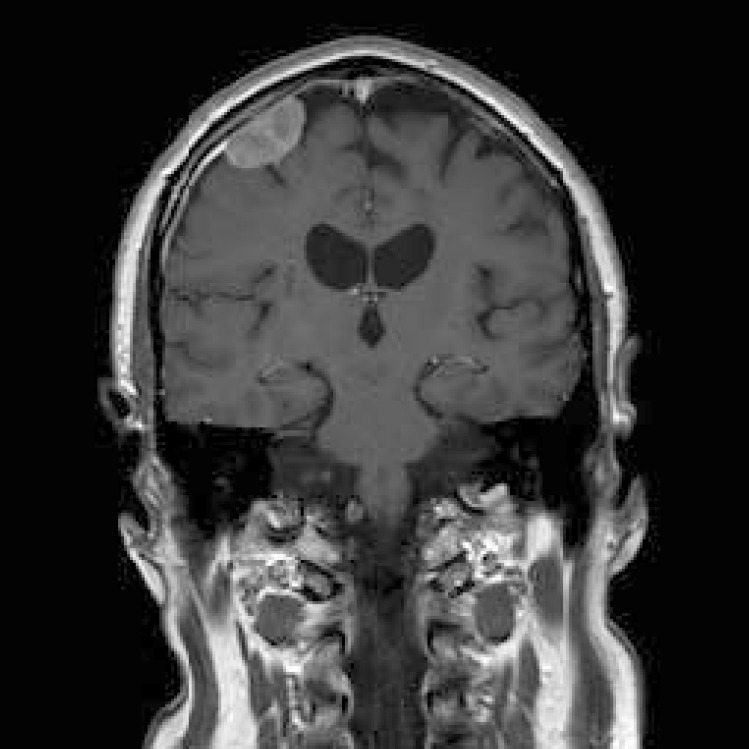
MRI (T1-weighted with gadolinium) at detection of seeded metastasis September 2009.
